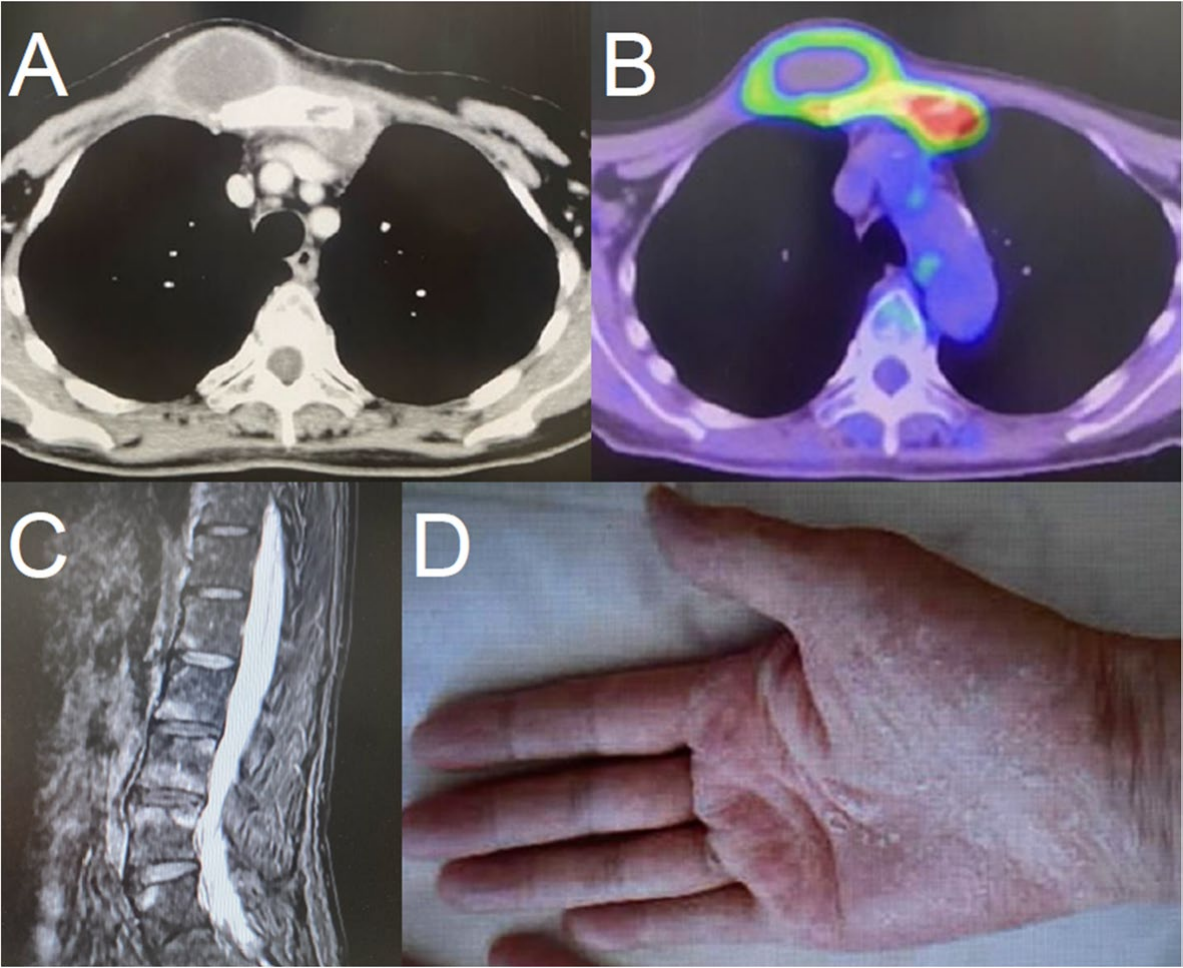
A, B CT and 18F-FDG PET/CT showing inflammatory cystic lesions measuring 49 × 45 × 27 mm at the right sternoclavicular joint and 26 × 17 × 9 mm at the mediastinal side of the left first rib joint. C, D Characteristic findings of SAPHO syndrome. C MRI showing patchy long T2 signals along the lumbar vertebrae. D Image showing palmoplantar pustulosis. CT, computed tomography; 18F-FDG PET/CT,18-fluorine-fluorodeoxyglucose positron-emission tomography/computed tomography; MRI, magnetic resonance imaging; SAPHO: synovitis-acne-pustulosis-hyperostosis-osteitis
